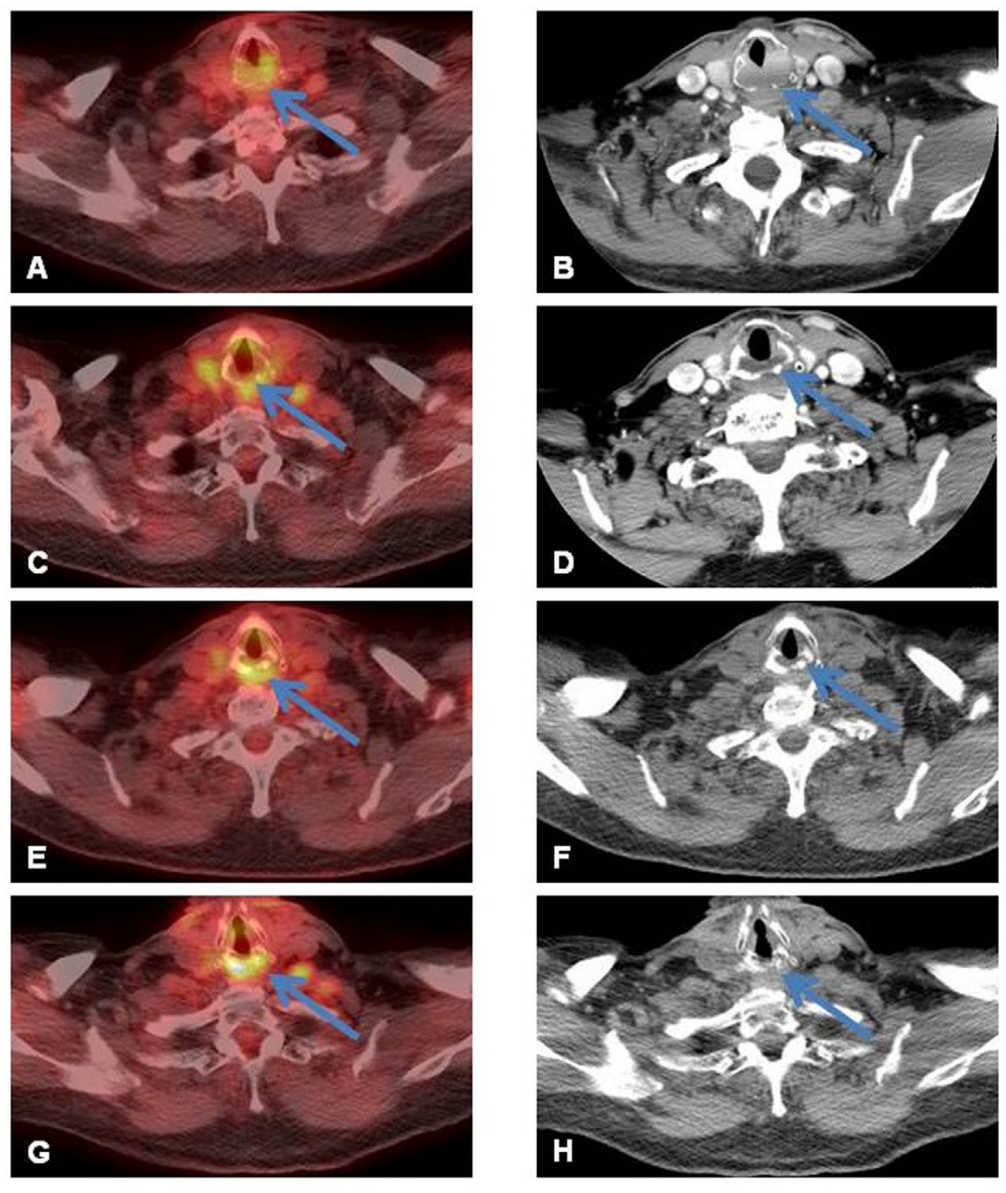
(A) Focal increased FDG uptake [maximum standard uptake value (SUV) of 3.8] in the larynx noted on axial fused PET/CT image (blue arrow) corresponds to a (B) soft tissue mass (3.3 cm × 1.7 cm × 2.2 cm) in the posterior cricoid cartilage on contrast-enhanced (CE)-CT scan of the larynx (blue arrow). (C) Posttreatment follow-up FDG-PET/CT acquired 2 months after the end of the radiation therapy showed a decrease of the intensity of uptake; persistence of a very low diffuse uptake (SUV 4.9) is noted probably reflecting post-radiation changes (blue arrow). (D) CE-CT of the neck confirmed decreased size of the residual lesion, now measuring 2.5 cm × 1.0 cm × 2.0 cm (transverse by AP by craniocaudal) (blue arrow) and improved narrowing of the infraglottic airway. (E) Follow-up PET performed 6 months later showed persistent non-specific uptake (SUV 4.7) and (F) essentially unchanged size of the residual soft tissue on non-contrast, low-dose CT of the PET/CT. (G) One-year follow-up PET/CT demonstrated non-specific uptake, and (H) on corresponding non-contrast, low-dose CT of the PET/CT, normal appearance of the cricoid without residual soft tissue was seen.
